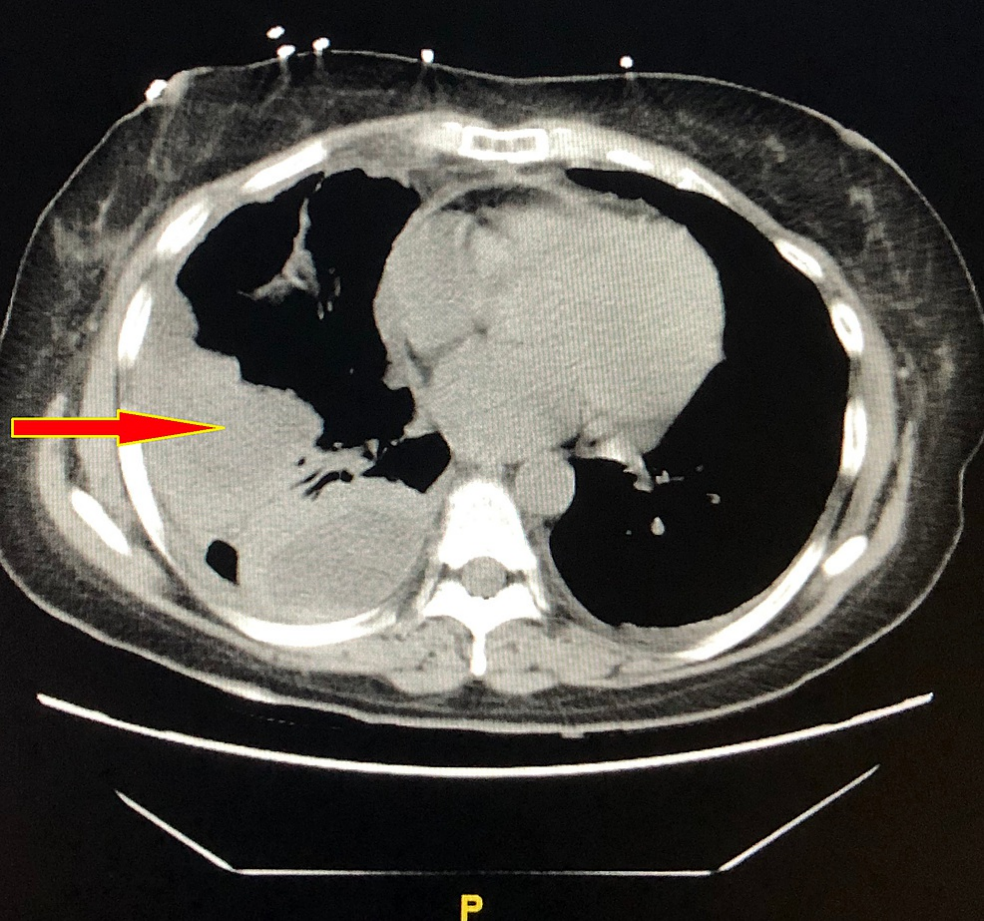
CT chest without contrast showing reaccumulating right-sided pleural effusion in comparison to Figure 3
